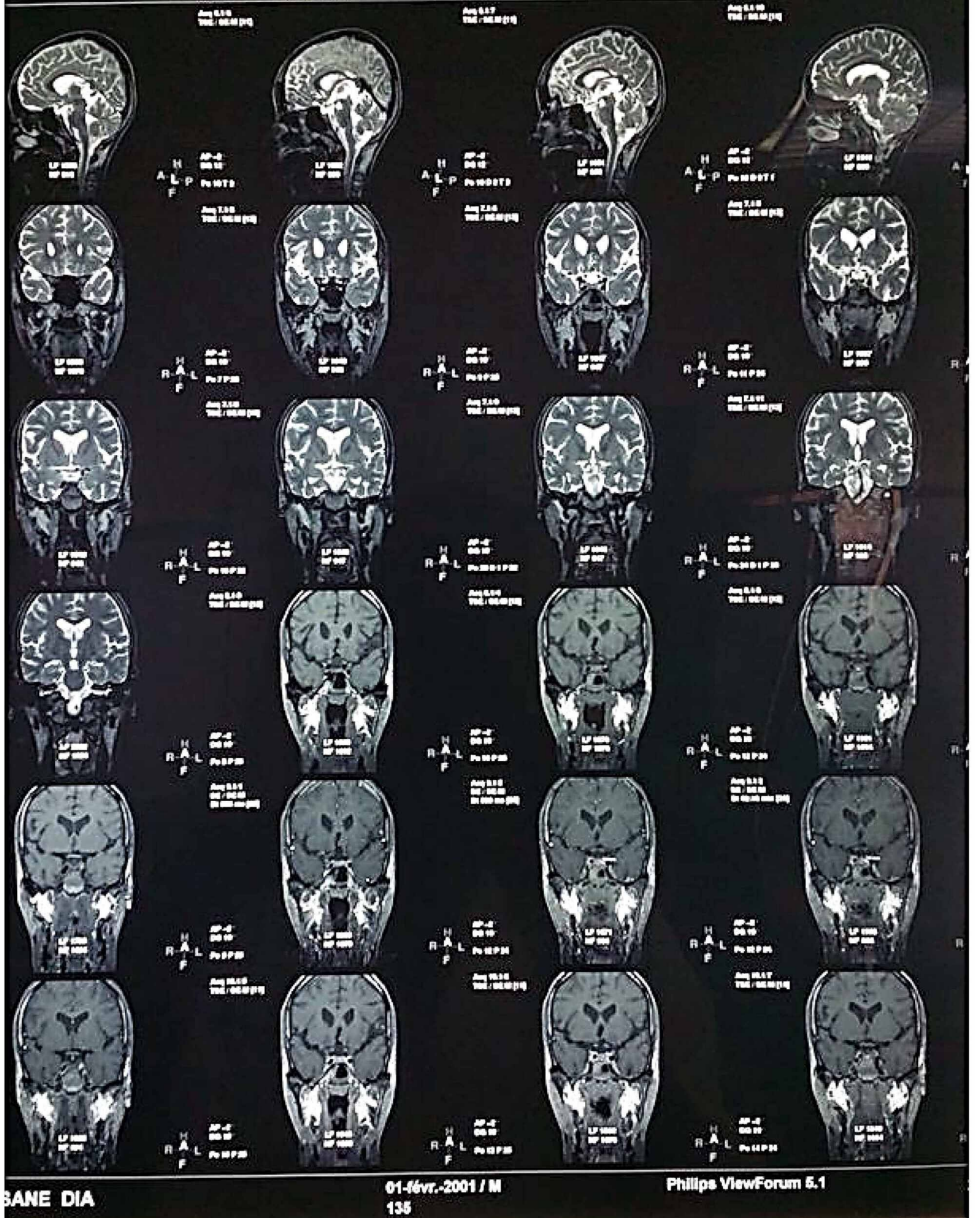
Cerebral magnetic resonance imaging of the patient
